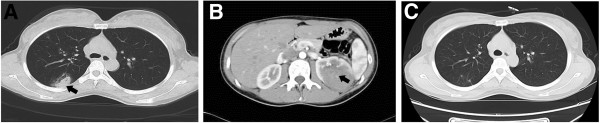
CT (computed tomography) imaging of the lung and kidney of the patient. Breast carcinoma with choriocarcinomatous features (BCCF) metastatic to the lung (A) and left kidney (B). The metastatic lesions are indicated with black arrows. (C) The lesions in the lungs disappeared after a further 9 cycles of capecitabine.
